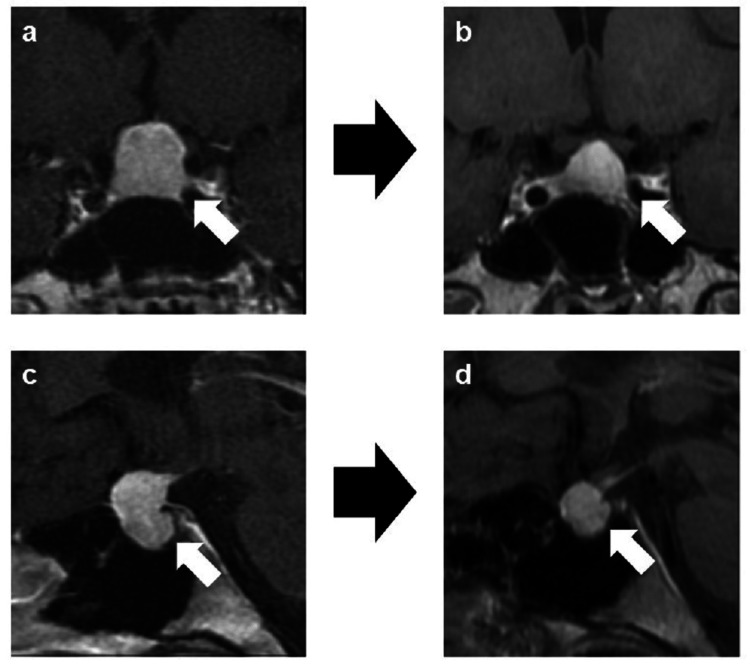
MRI scan imaging of the pituitary gland. Coronal contrast-enhanced T1-weighted (a, b) and sagittal contrast-enhanced T1-weighted (c, d) MRI scans at the first visit to our hospital (a, c) and 10 months after administration of levothyroxine (b, d). After 10 months of levothyroxine replacement therapy, a follow-up MRI showed dramatic pituitary shrinkage of approximately 50% to a tumor size of 16.79×11.28×12.59 mm (white arrows). MRI: magnetic resonance imaging
